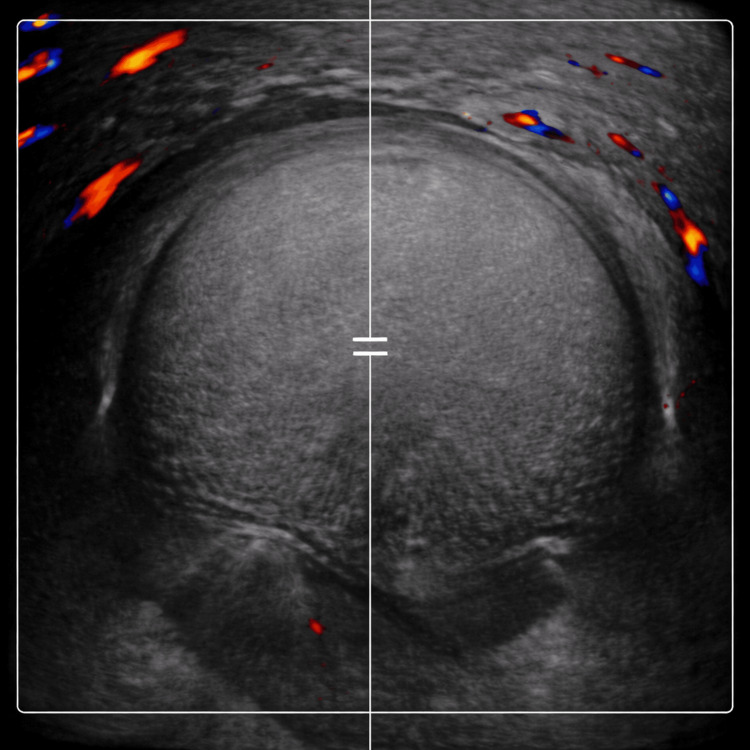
Transverse color Doppler ultrasound of the mid-right testicle demonstrating a complete absence of internal macrovascular flow. While peripheral blood flow is noted in the surrounding scrotal wall, the lack of intratesticular signaling is a hallmark finding of high-grade testicular torsion and global ischemia.
